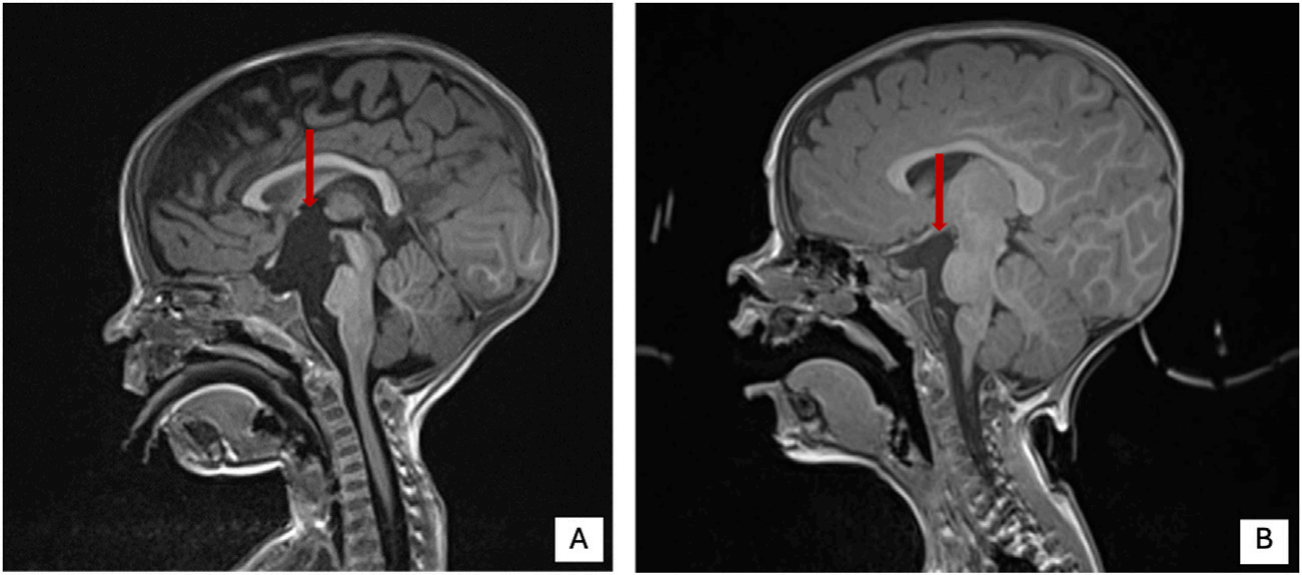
Brain MRI pre and post-surgery in patient 1 of present cohort. (A) Brain MRI pre surgery. (B) Brain MRI post-surgery. Red arrow shows the cystic lesion.
